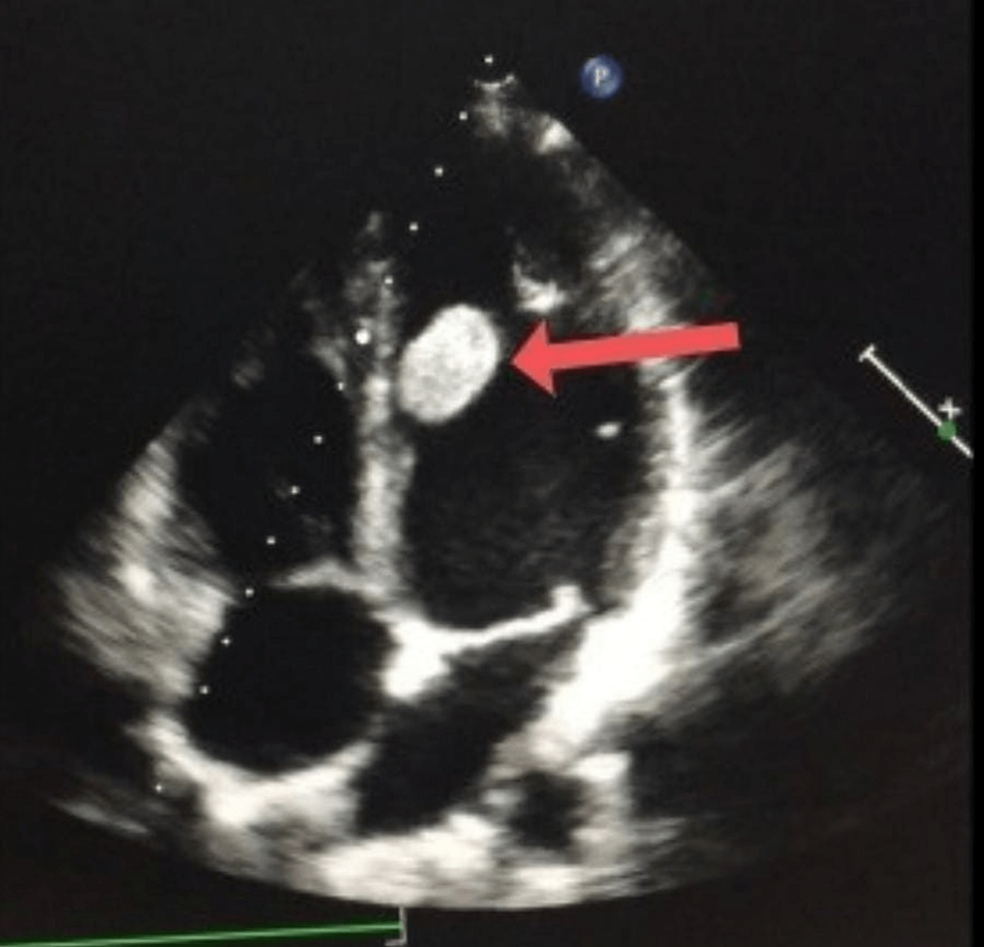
Echocardiography showing a 2x2 cm encapsulated tumor attached to the mid-septum below the aortic annulus.
